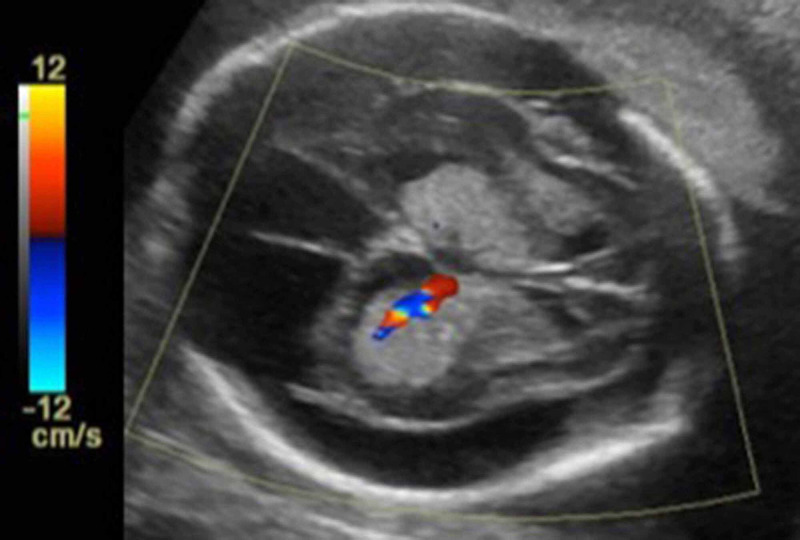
Follow-up color Doppler ultrasound at 22 weeks 6 days, obtained by transabdominal ultrasound, shows progressive ventriculomegaly with notably enlarged extra-axial spaces There is increased vascularity of the echogenic choroid plexus. This finding helps corroborate that the enlargement is due to choroid plexus papillomas, rather than intraventricular hemorrhage.
